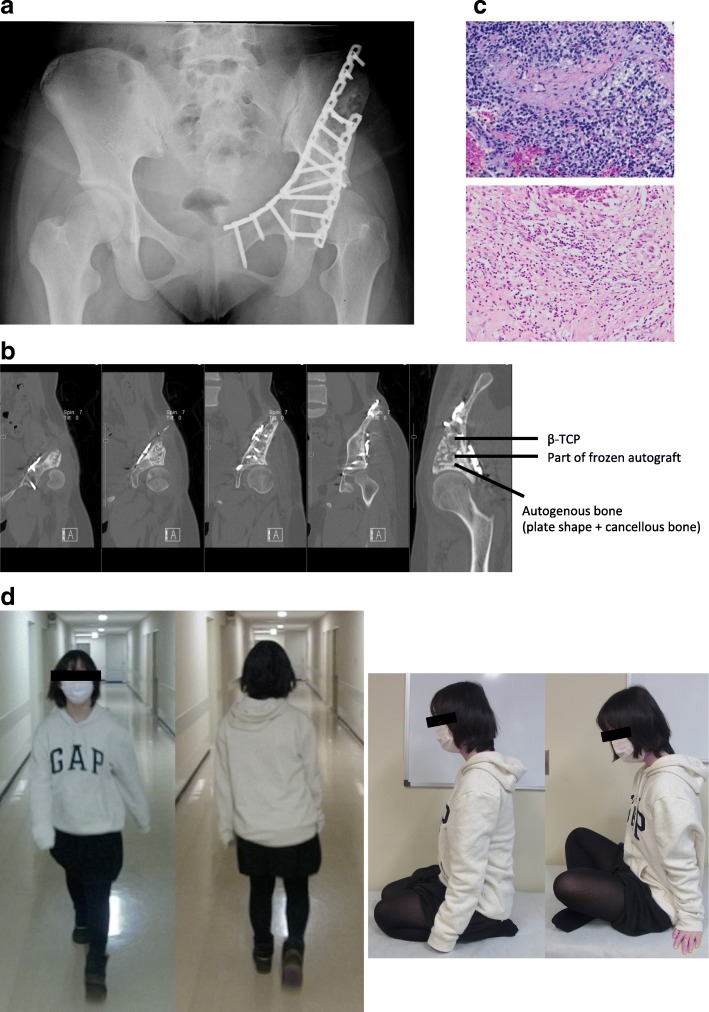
Postoperative assessments in case 1: A 12-year-old girl with Ewing sarcoma of the pelvis (PI-II). a Radiograph obtained at 23 months after reconstruction. b Computed tomography at 23 months after reconstruction. Bone union is observed, and osteoarthritis is not yet observed. c Pathological findings at biopsy (upper panel) and post-neoadjuvant chemotherapy (lower panel). After neoadjuvant chemotherapy, a necrotic change was observed. d She can walk almost normally, and there are no limitations in the sitting posture
